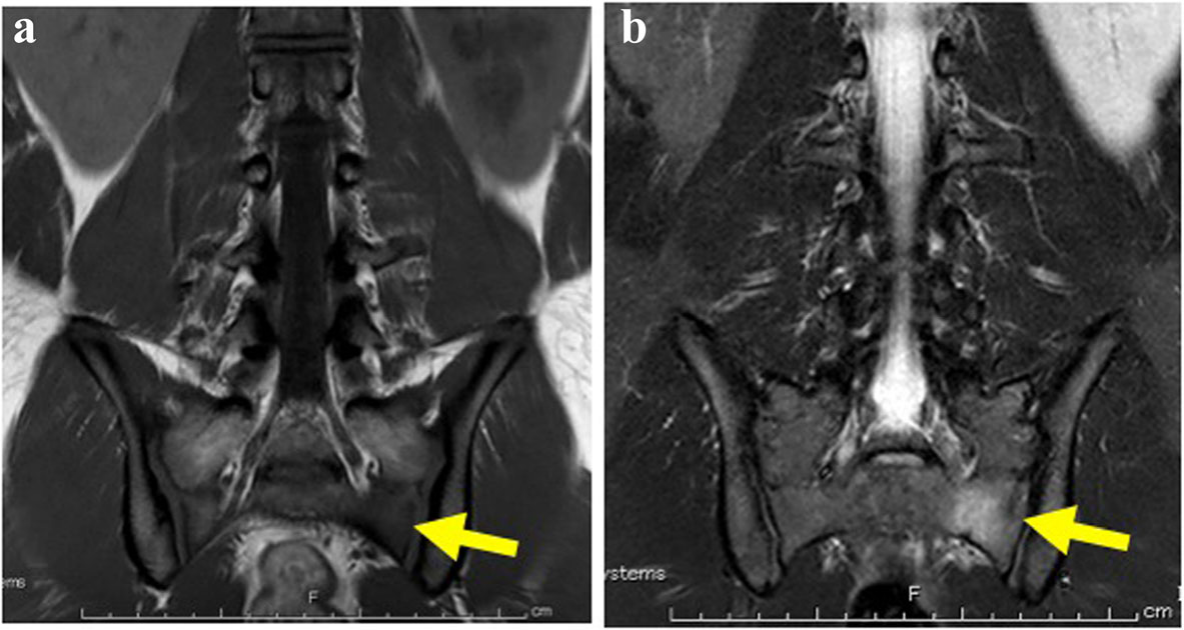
Coronal T1-weighted (a) and short inversion time inversion recovery (b) images of the pelvis onsecond presentation. Bone marrow edema in the left sacral ala (*arrow*) indicates left sacral fracture
